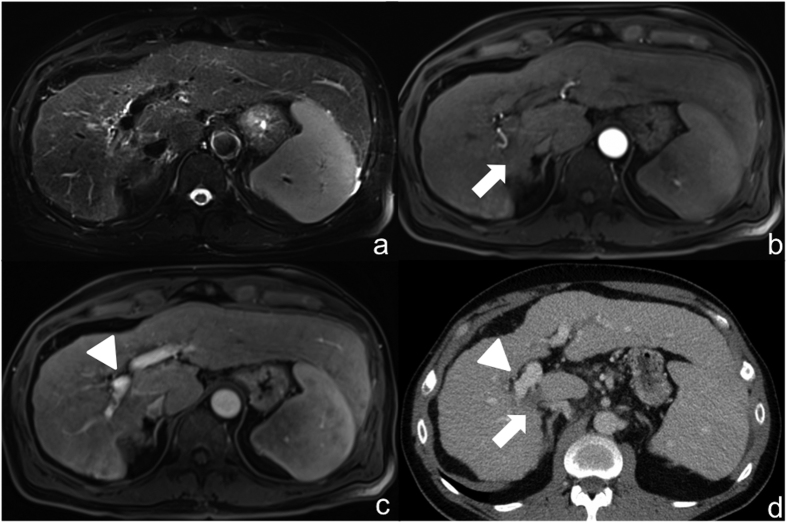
Same patient as in Figs 1, 2, 3 and 4: Follow-up 2 years post-intervention: (a) Native, fat-saturated T2 sequence: cicatricial changes after IRE ablation with distinct shrinkage of the ablation defect. (b) Dynamic, fat-saturated T2 sequence after contrast: in arterial phase no indication of arterial hypervascularization (arrow), no indication of recurrence. (c) Dynamic fat-saturated T1 sequence after contrast: in portal venous phase continued full contrast of portal vein (tip of arrow). (d) Follow-up CT in the portal venous phase with full contrast of portal vein (tip of arrow) and distinct shrinkage of the hypodense ablation defect 2 years post-IRE (arrow).
